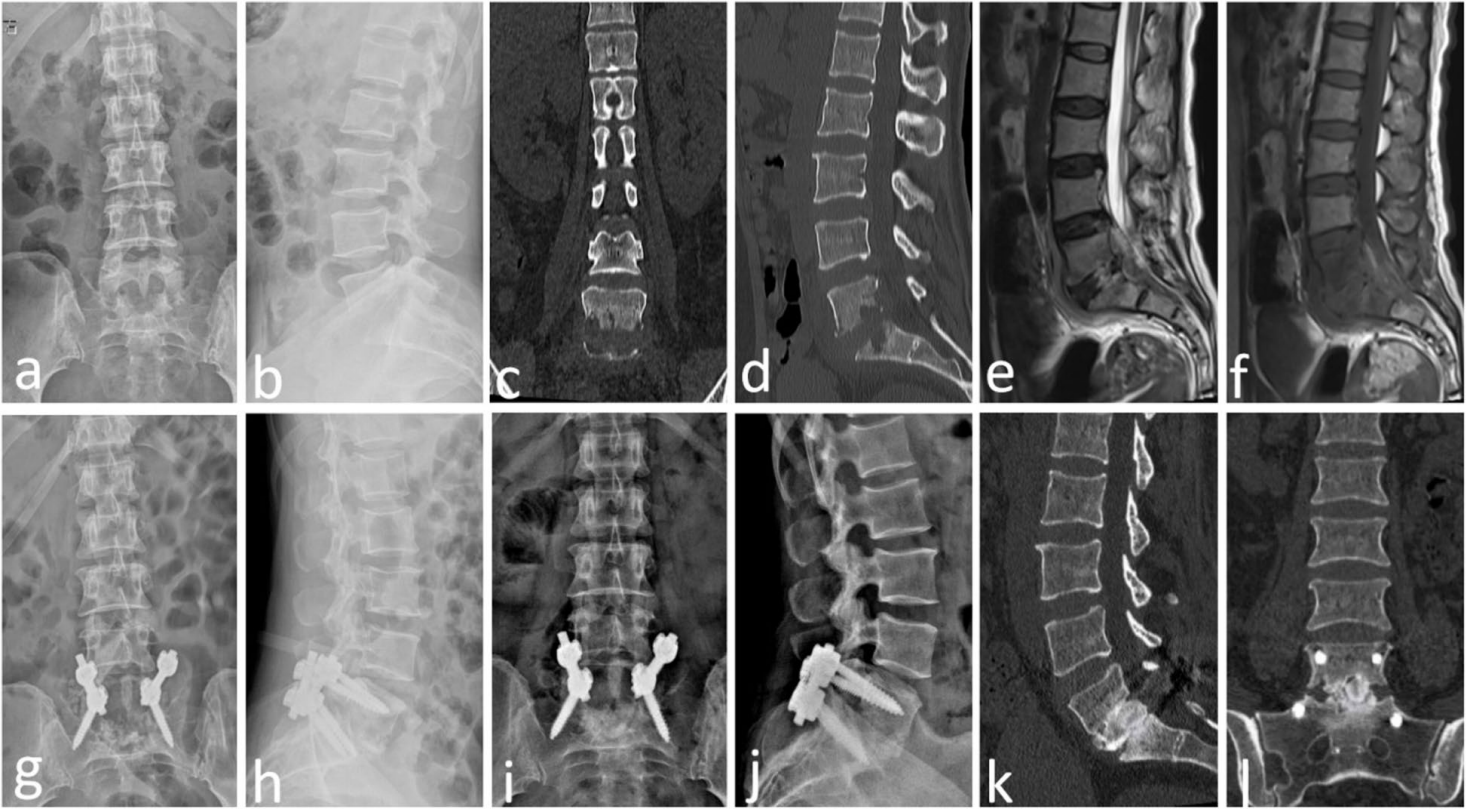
Case No. 2. A 52-year-old female underwent one-stage posterior surgery of L5-S1 due to a chronic E. coli infection. S1 alar screws were applied in single-segment lumbosacral fusion and fixation. (a–f) Preoperative X-ray, CT and MRI scans showed bone destruction with significant vertebral body loss at L5 and S1. (g, h) Postoperative X-ray images 4 days after the operation showed signs of posterior fusion and fixation of L5-S1 and autologous grafting, screws and rods in good location. (i–j) X-ray images 6 months after surgery showed partial bone fusion between L5 and S1. (k–l) CT images 12 months after surgery showed solid bone fusion between L5 and S1
